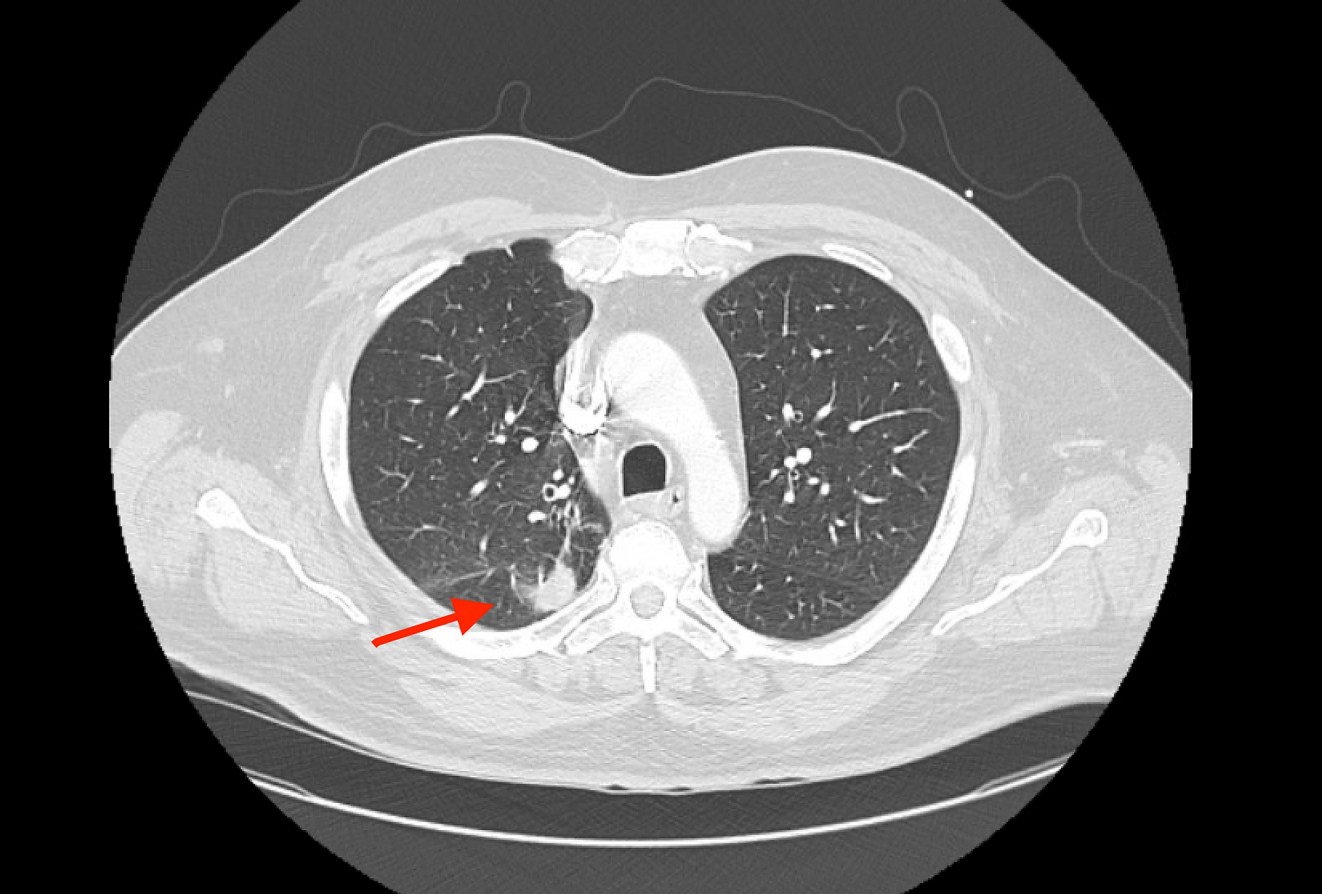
CT of the chest without significant findings, besides a small, linear right upper lobe infiltrate (red arrow).
